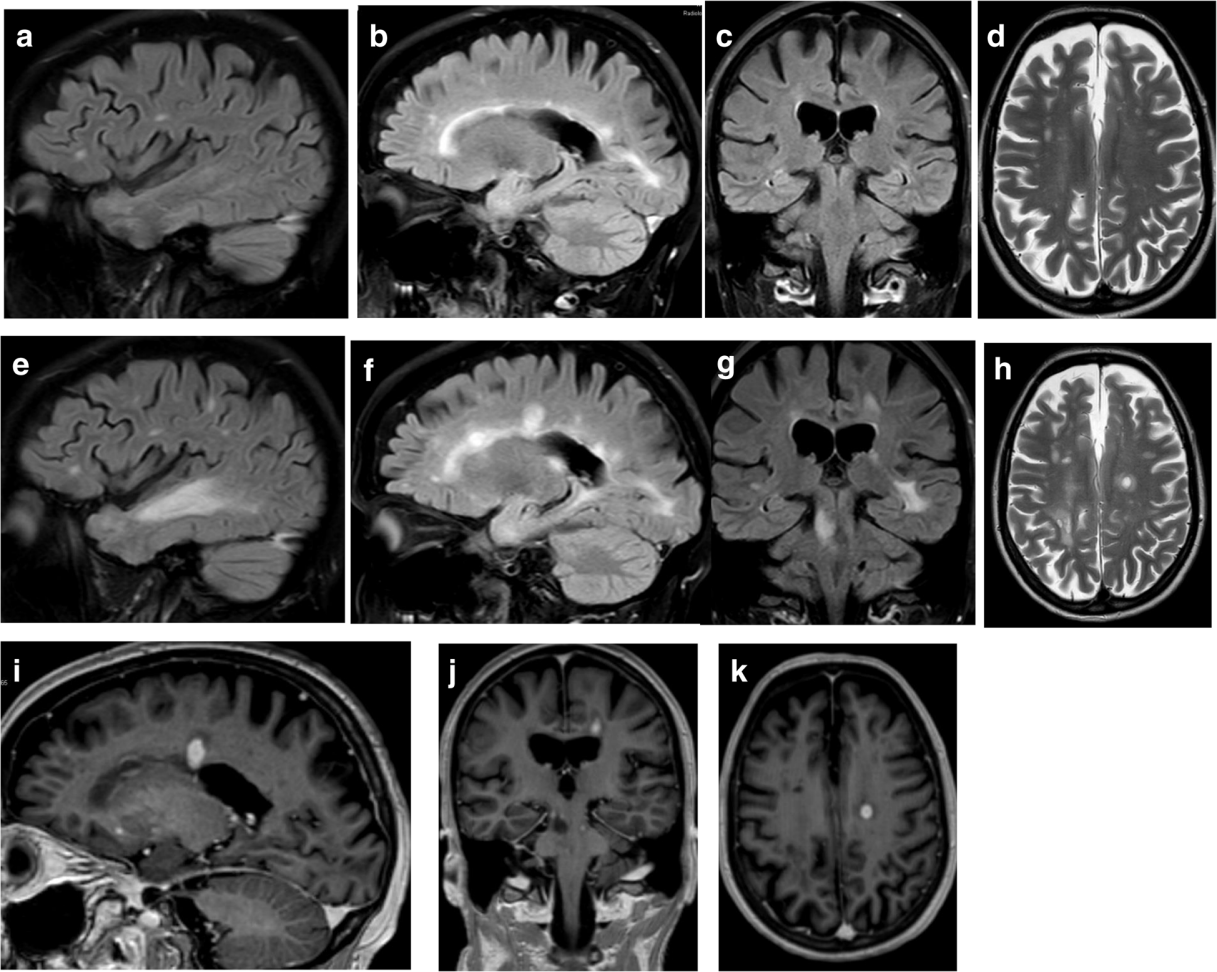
MRI during the early phase and at the peak of post-natalizumab exacerbation. The upper panel shows Fluid inversion recovery (FLAIR; a, b and c) and T2 (d) from June 2015. The middle panel shows examples of new and enlarging lesions on corresponding FLAIR (e, f and g) and T2 (h) sections at March 2016. The lower panel (i, j and k) shows examples of gadolinium enhancement on T1 images at March 2016
